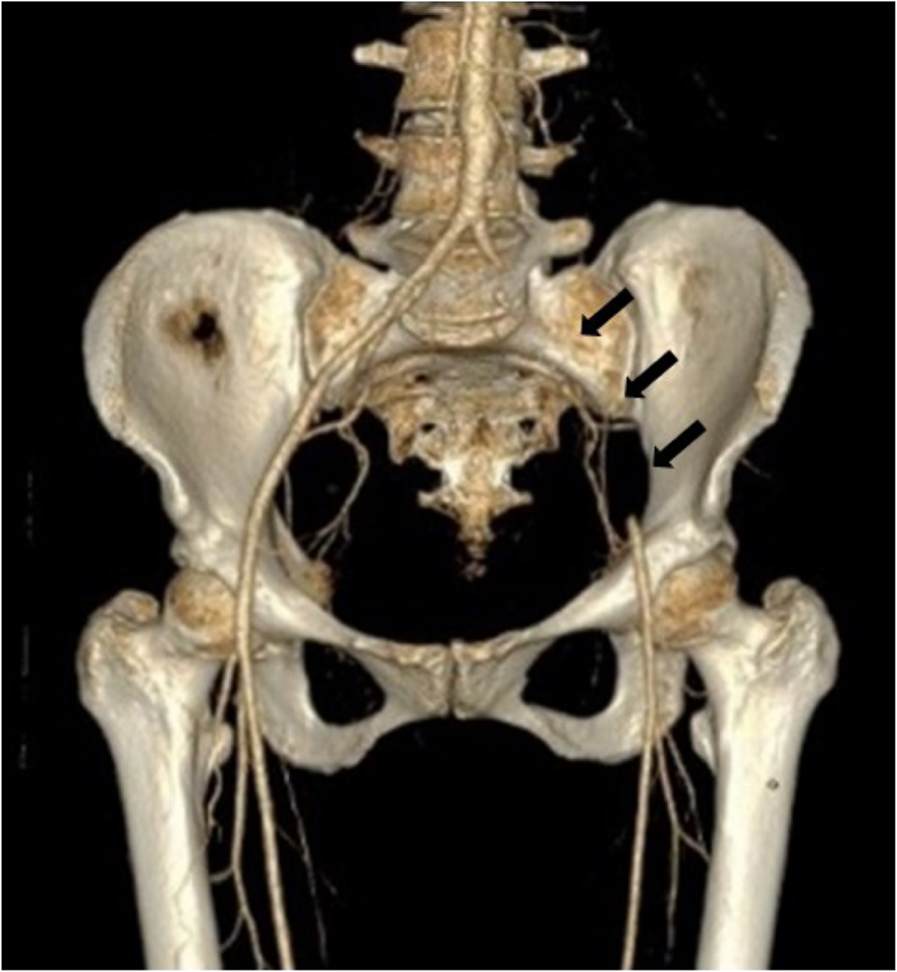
Computed Tomography Angiography showing occlusion of the left sided iliac artery distal of the aortic bifurcation (triple arrow)
